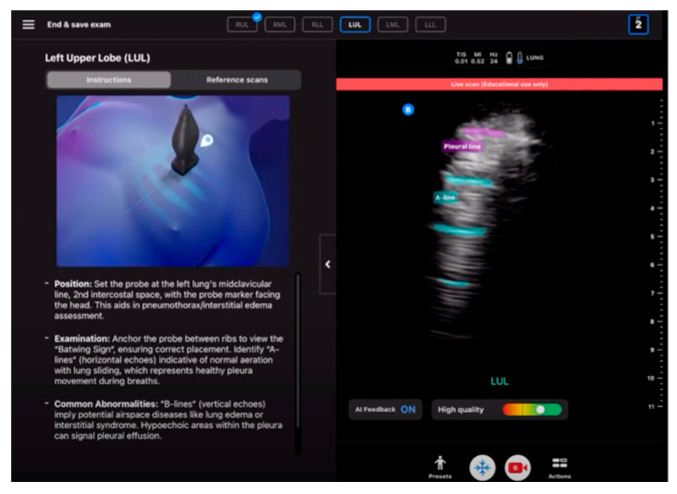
Automatic detection of A-line in lung ultrasound, adapted from (https://www.butterflynetwork.com/iq-plus) with permission publisher 2024 (accessed on 1 January 2024) [29].
